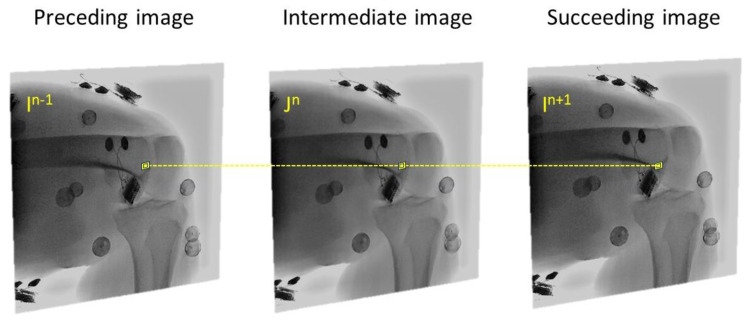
The intermediate image (*J*^*n*^) at time point n of B X-ray unit could be reconstructed from the preceding (I^*n*−1^) and succeeding image (I^*n*+1^) using the motion-compensated frame interpolation method.
